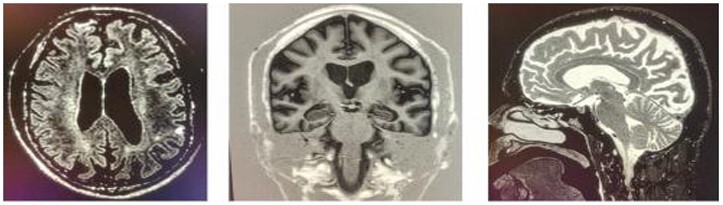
Brain magnetic resonance images of patient GLS, in which we can see cortical atrophy in the frontal lobe, as well as a reduction in global brain mass.
